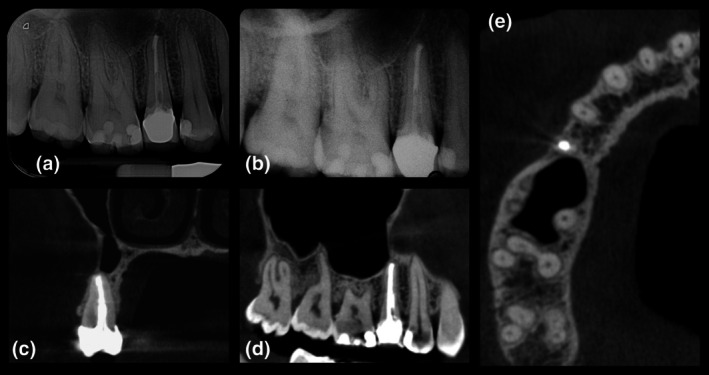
(a) Immediate post‐operative baseline; (b) one‐year follow‐up intraoral radiograph; (c–e) CBCT at 1 year, coronal, sagittal, horizontal axial view showing complete healing of the lesion and maxillary sinus.
